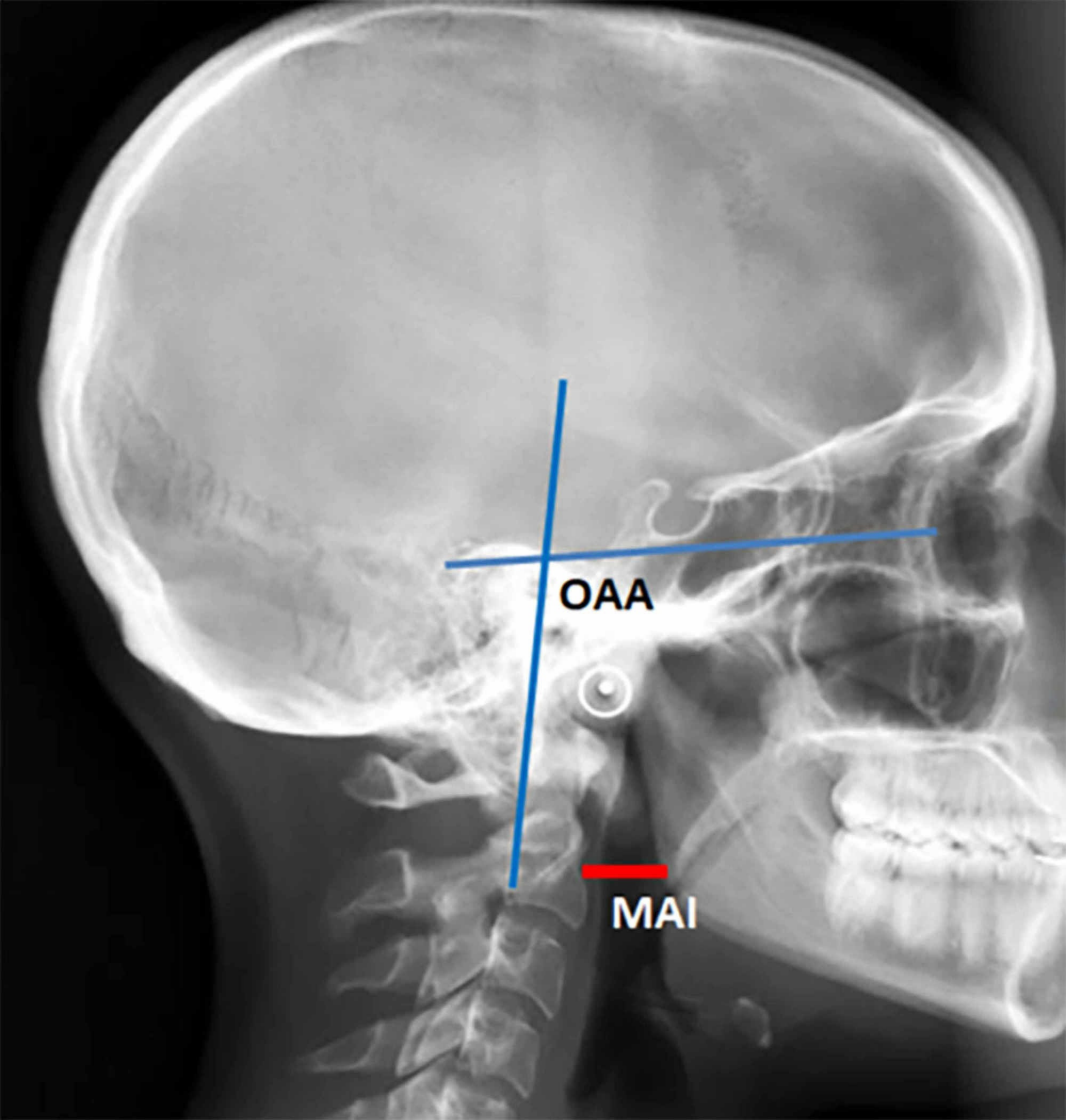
Orbital-axial angle and mandible-axial interval marked on a lateral skull X-ray The orbital-axial angle (OAA) is that angle subtended by a line from the mid-orbit tangential to the base of the pituitary fossa and the posterior axial line. The OAA should approximate 100° (95-105°). The mandible-axial interval (MAI) is a direct measure of the retropharyngeal space, optimally between 10 and 20 mm
